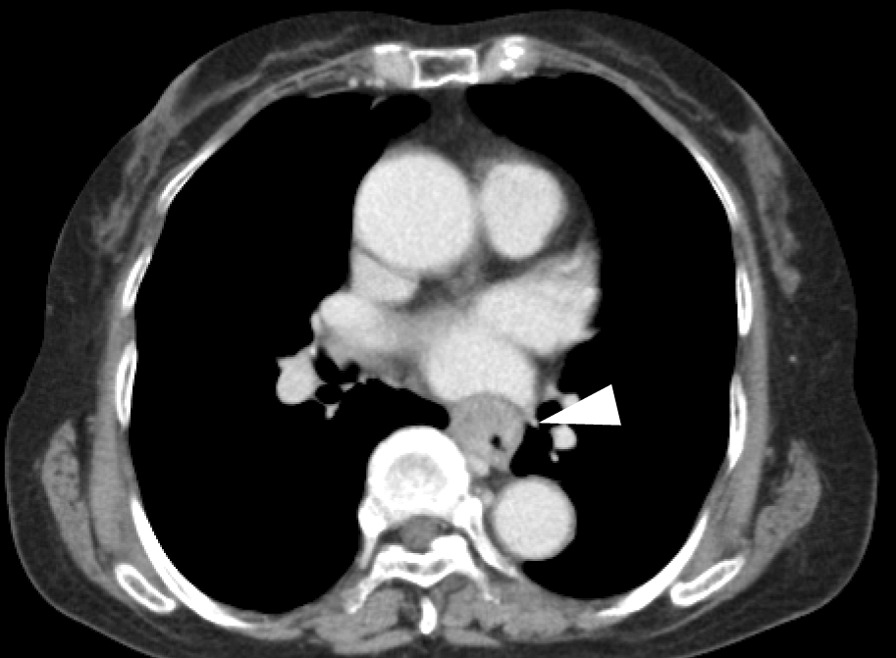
Enhanced computed tomography revealing wall thickening measuring 30 mm in size in the middle-third of the intrathoracic esophagus (arrow head), with no significant lymph node or distant metastasis
